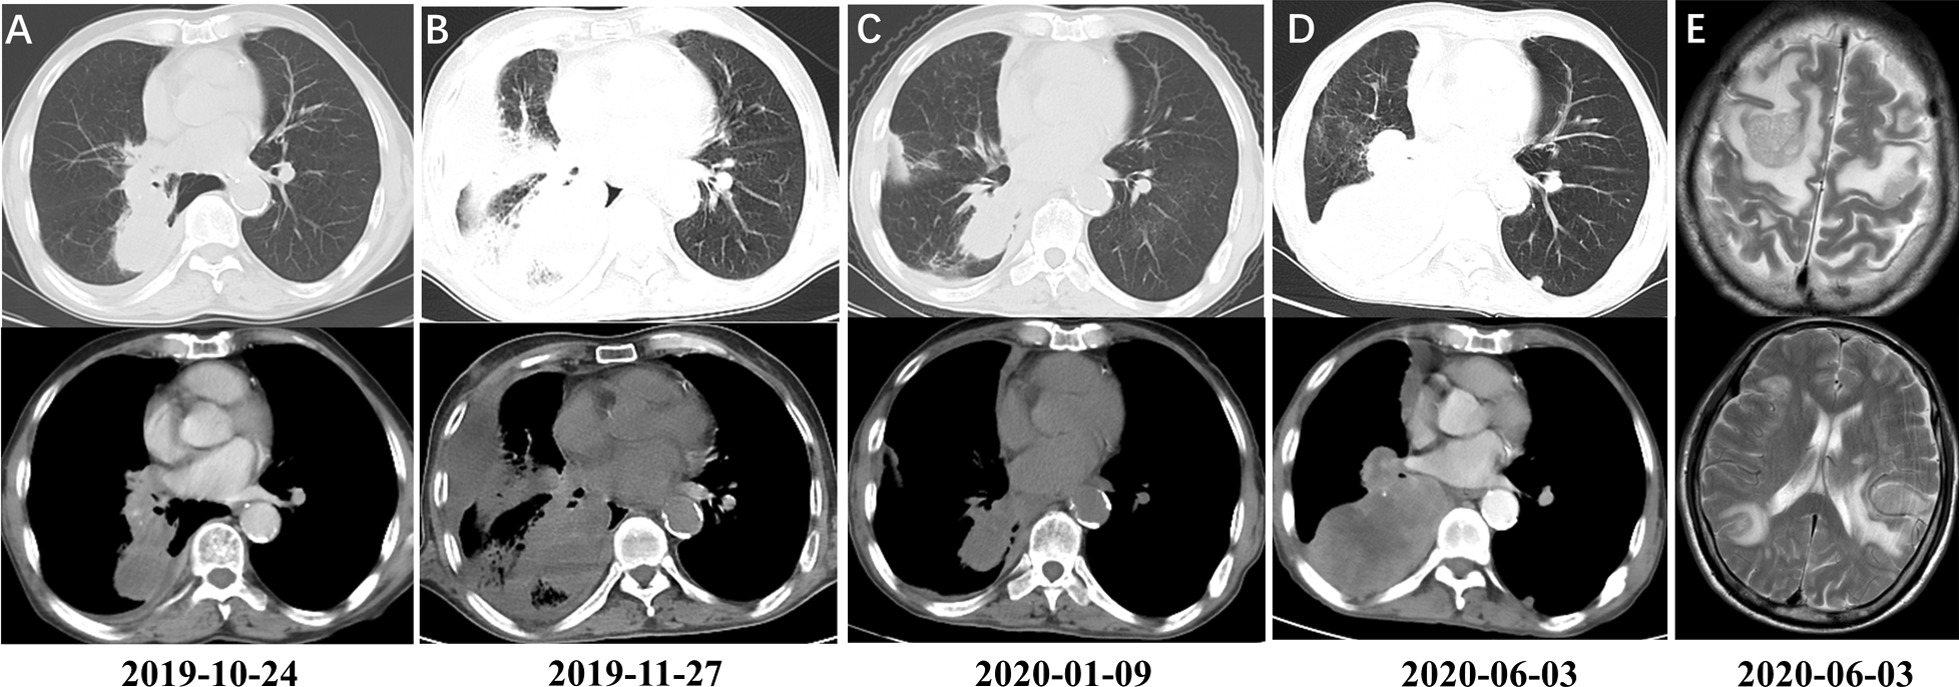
Dynamic imaging of lung lesions at different stages of Crizotinib treatment. A The baseline of diagnosis (before treatment): Chest computed tomography scan revealed a mass in the right middle and lower lobe (3.6*3.4 cm), and enlarged lymph nodes in the right hilum and mediastinum; B The first evaluation after one cycle of chemotherapy showed progressed of disease with enlarging lymph nodes in the right hilum and mediastinum; C The second evaluation after 1 month of Crizotinib therapy showed partial remission according to RECIST1.1 criteria; D and E Evaluation after approximately 6 months of Crizotinib treatment showed progressive disease of lung lesion and metastasis in right pleura and brain. The measuring scale of all CT image is 20 cm (equipment: GE optima 2), and the MRI is 10 cm (equipment: Philips multiva 1.5 T)
